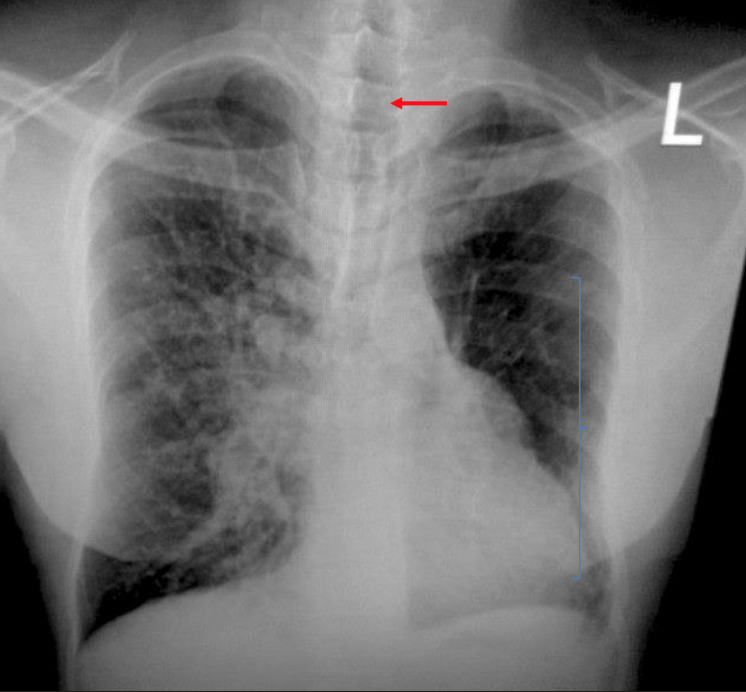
Chest X-ray showing reduction in the size of the left hemithorax, plethora of the right lung and leftward shift of the mediastinum.
